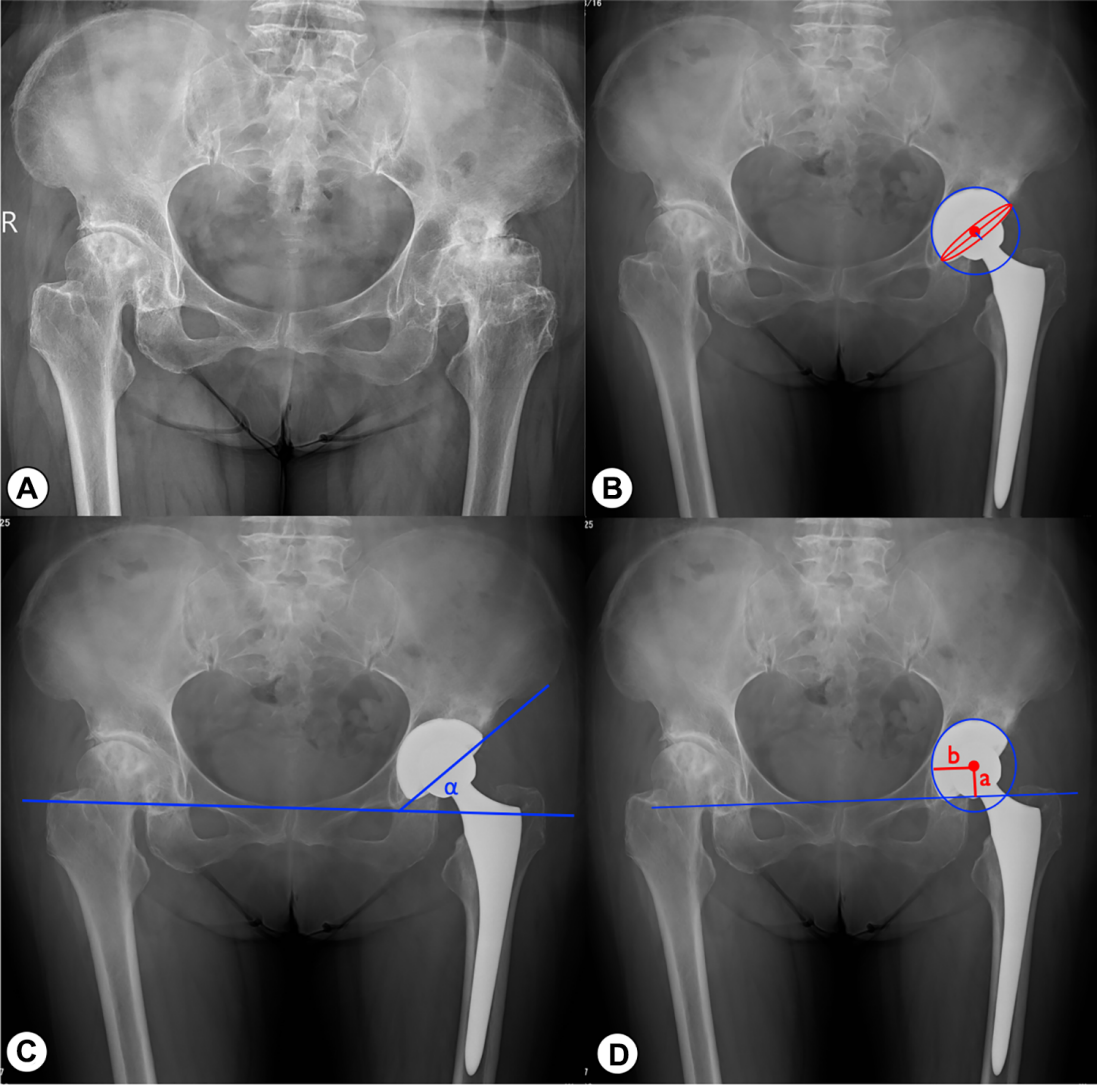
Imaging measurements on postoperative anteroposterior X-rays. (A) Preoperative radiograph of a patient with bilateral DDH. (B) Measure-ment of acetabular anteversion. Anteversion angle = arcsin(a/b); a is the minor axis of the red ellipse (the blue straight line in the figure); b is the major axis of the red ellipse (the red straight line in the figure). (C) Measurement of acetabular abduction angle. (D) The vertical rotation center (a) and the horizontal rotation center (b) of the hip joint.
